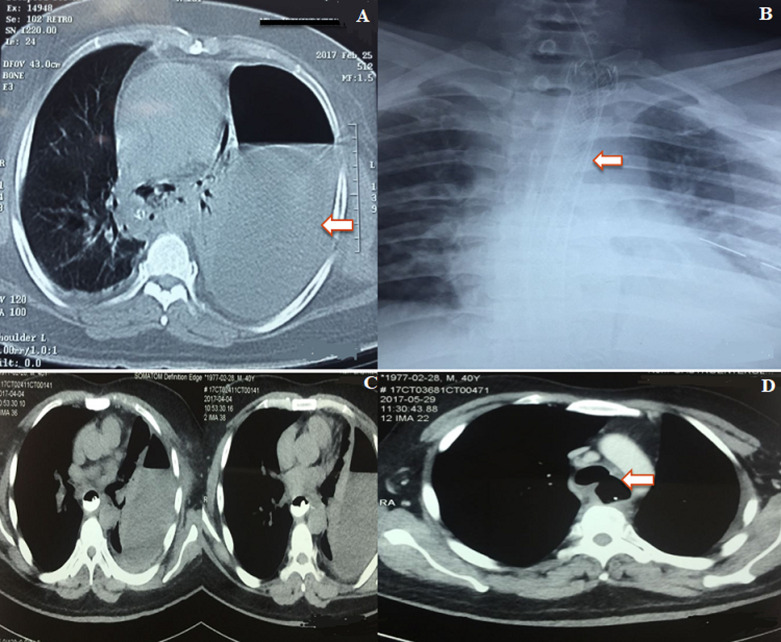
A) CT chest showing left pleural effusion (white arrow) post esophageal rupture; B) chest x-ray showing endoscopically placed SEMS in situ; C) post stent removal, CT thorax showed a 3mm esophagopleural fistula from the distal esophagus to the left pleural cavity; D) post repeat stenting and stent removal, CT thorax showed tracheoesophageal fistula (arrow) and no leak from the distal esophageal perforation
